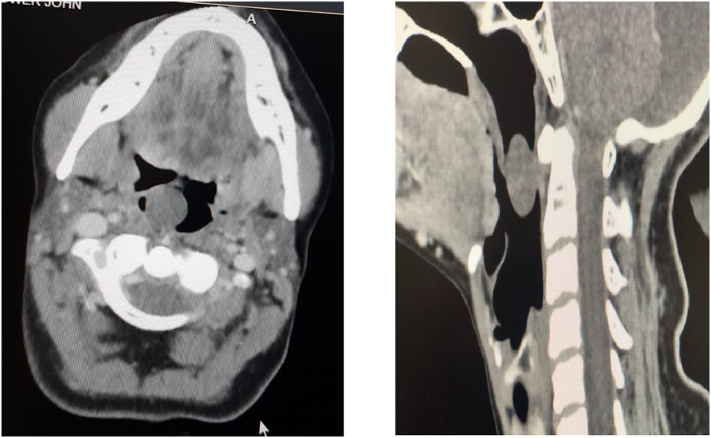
Axial and sagittal CT scans showing a soft tissue lesion arising from the right side of the soft palate.
